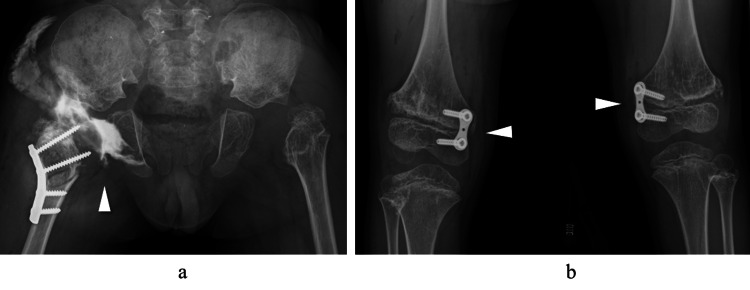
Postoperative plain radiographs. (a) Anteroposterior view of the hip: The femoral head remains reduced even in slight abduction of the hip (white arrowhead). (b) Anteroposterior view of the knee: Guided growth was performed using a tension band plate on the distal medial femur (white arrowheads).
